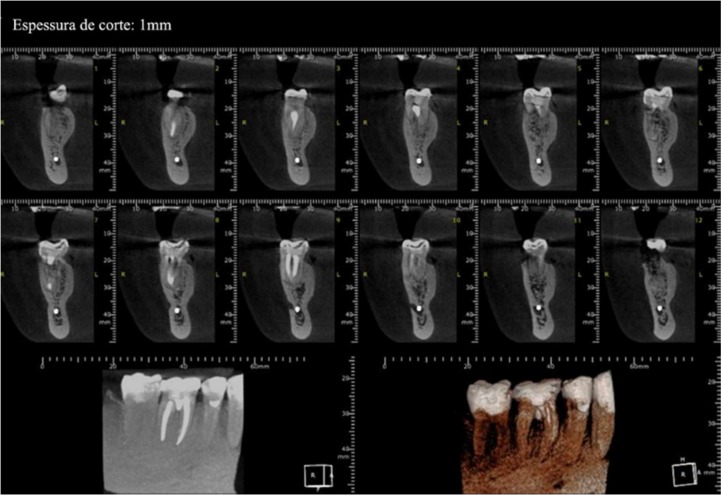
Cone-beam computed tomography images and periapical radiography after 7-year follow-up
